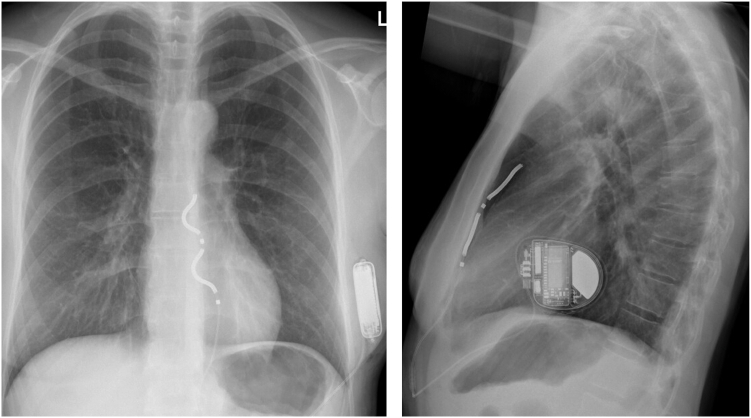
Postoperative chest radiograph of case 2. After an uneventful implantation, the extravascular implantable cardioverter-defibrillator lead demonstrates a tilt toward the heart.
